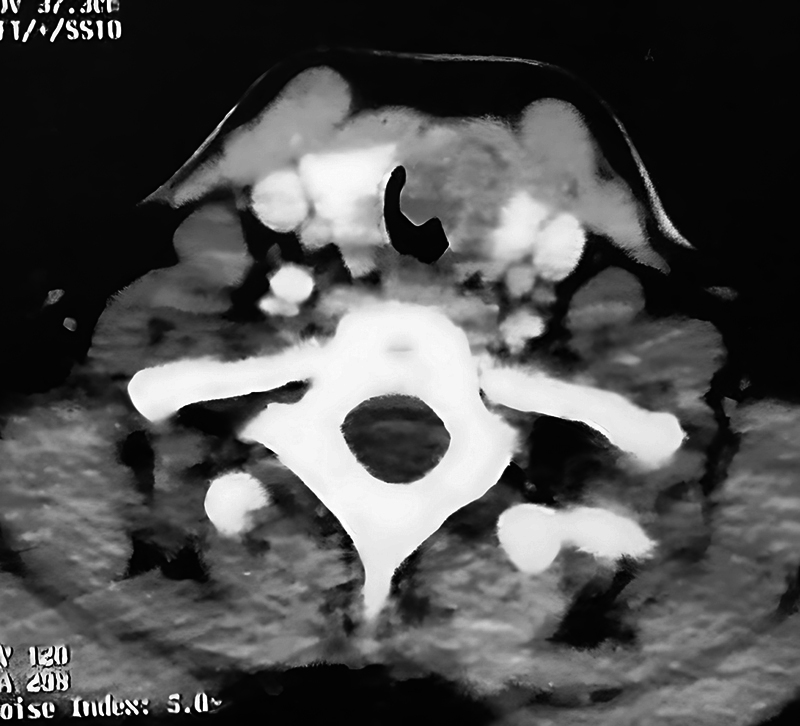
Computerized tomography (CT) scan showing a thyroid gland tumor infiltrating the trachea.
